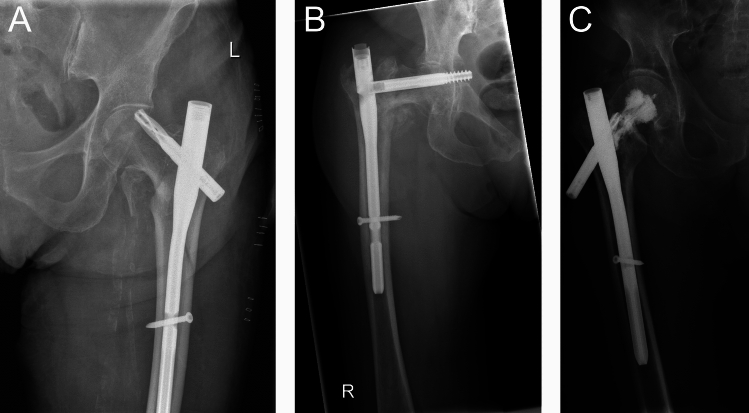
Representative radiographic examples of mechanical complications following intramedullary nailing of proximal femoral fractures. A Cut-out: Cranio-lateral penetration of the blade or screw through the femoral head after implantation of a cephalomedullary nail. B Cut-in: Central or superomedial migration of the blade or screw into the acetabulum, or in this case, even extending into the lesser pelvis. C Lateral protrusion: Lateral migration of the blade or screw beyond the greater trochanter
